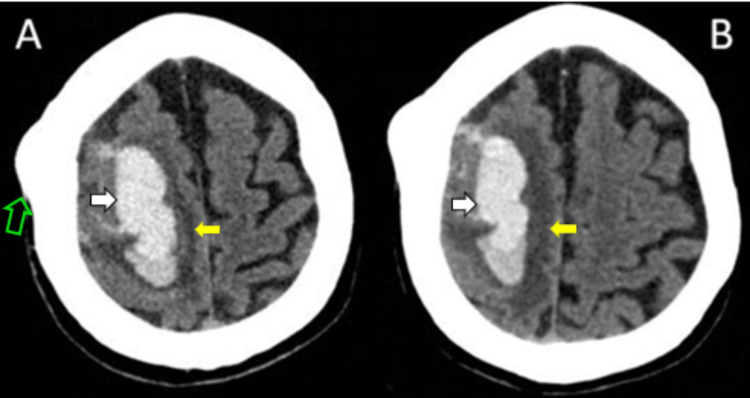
(A) Initial axial CT scan of the head showing right frontal hemorrhage (white arrows) with a surrounding hypodensity suggestive of a perihemorrhagic edema (yellow arrows). (B) After 48 hours, an increase in perilesional edema was noted. An osteoma in the right frontal bone is also seen (green arrow). CT: Computed tomography
